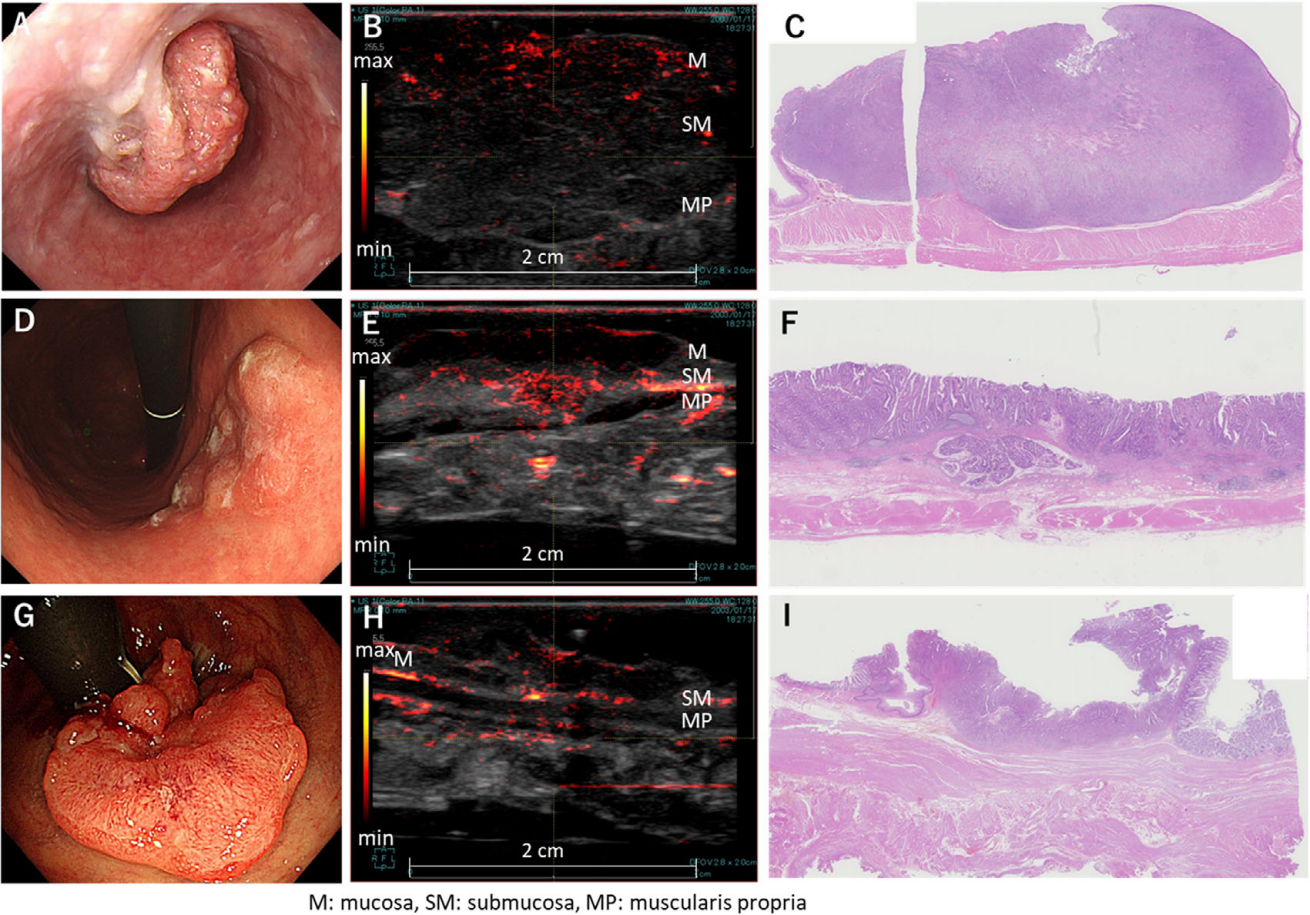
Photoacoustic (PA) image of T1 gastrointestinal cancer. PA images are superimposed on gray‐scaled B‐mode ultrasound (US) images in (B), (E), and (H). The color bars indicate the PA image intensity. (A) Esophagus, 30 mm, Is, pSM3, (B) photoacoustic image of the deepest invaded area of cancer, (C) pathological image of the same site as the photoacoustic image, (D) stomach, 40 mm, IIa, pT1b, (E) photoacoustic image of the deepest invaded area of cancer, (F) pathological image of the same site as the photoacoustic image, (G) rectum, 25 mm, IIa+IIc, pT1b, (H) photoacoustic image of the deepest invaded area of cancer, and (I) pathological image of the same site as the photoacoustic image
